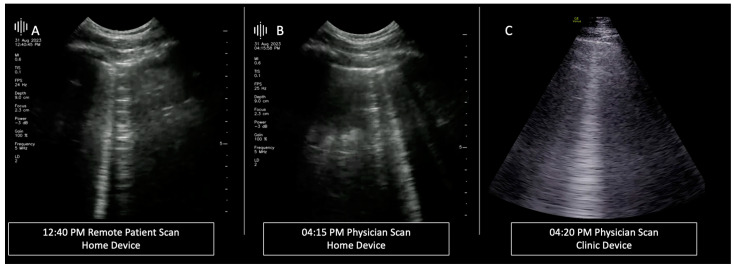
Scan Flow. A sequence is depicted of dialysis ultrasound scans. (A) shows a remote patient scan conducted at 12:40 PM using the home device. (B) presents a scan performed by a physician at 04:15 PM using the same home device. (C) provides a comparison with a physician’s scan at 04:20 PM using the clinic device, showcasing the benchmark quality for lung ultrasound images.
